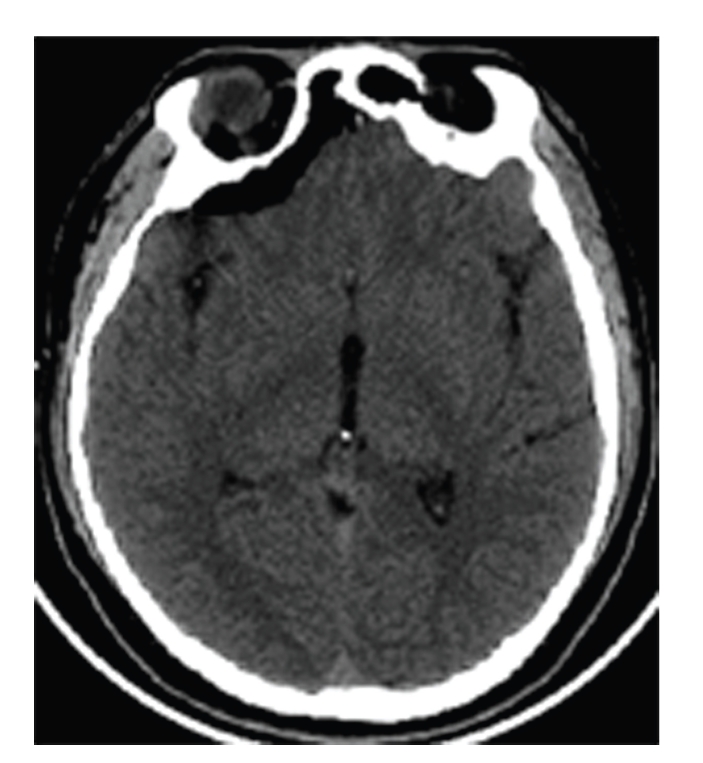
Head CT without contrast demonstrating complete removal of cyst.
